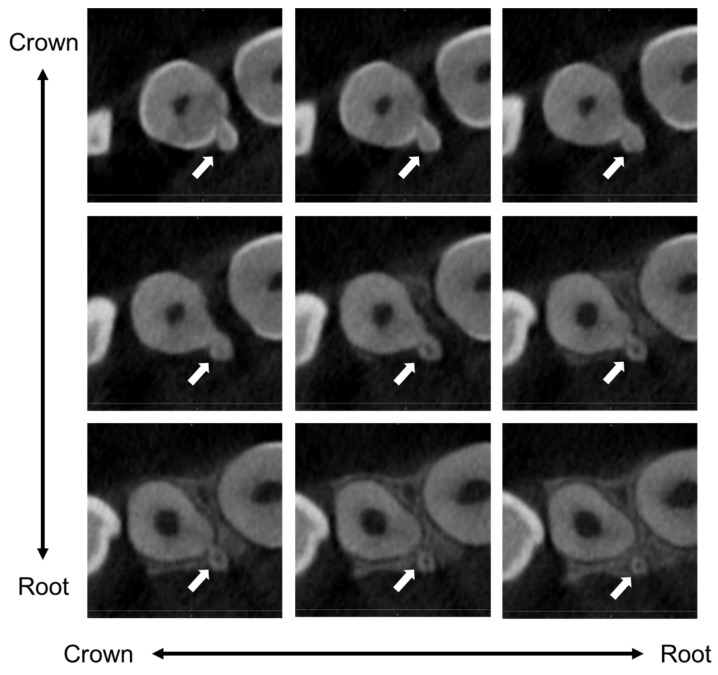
Cone-beam computed tomography imaging of a horizontal section at the age of 11 years and 6 months. The calcified tissue had a crown, root, and pulp chamber; was independent of the maxillary right lateral incisor; and was diagnosed as a supernumerary tooth. Arrows indicate the tooth fused with the supernumerary tooth.
